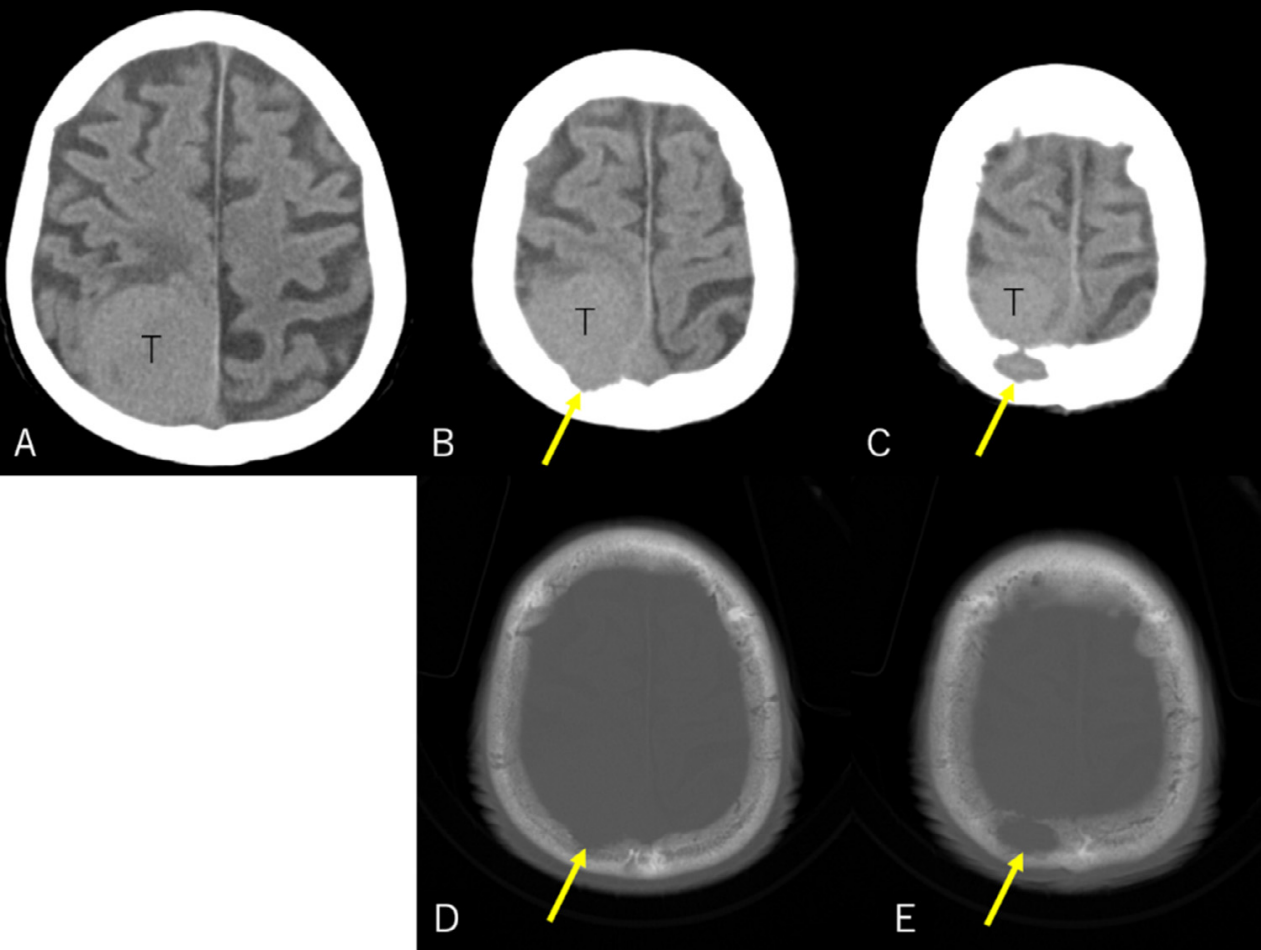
Noncontrast axial computed tomography scans (*A-C*) and relevant bone-target images (*D, E*) showing an isodense mass in the right parietal lobe with a smooth-contoured focal erosion in the adjacent parietal bone (*B-E,* arrow). Neither intralesional calcification nor hyperostosis are observed in the adjacent skull. T: tumor. *A* (inferior)→*C* (superior).
